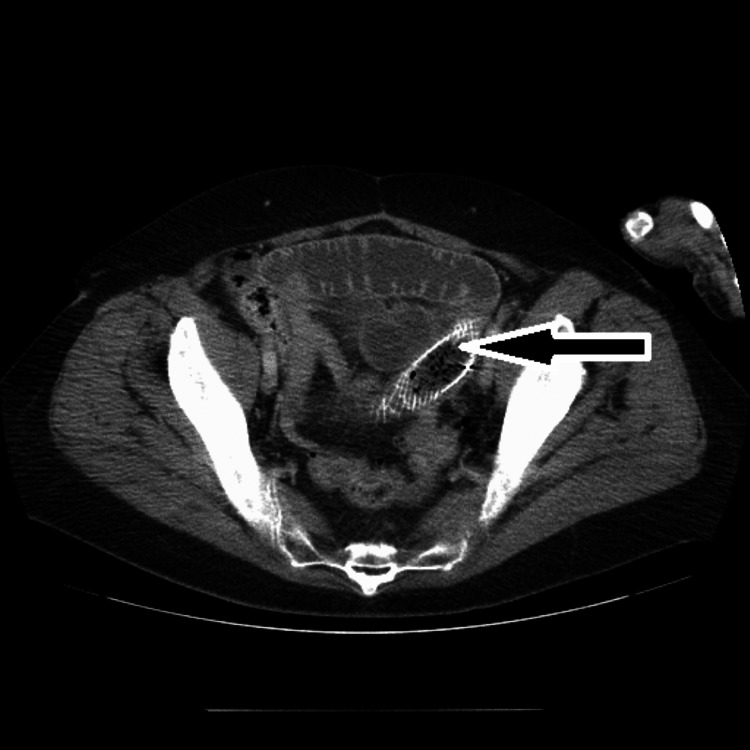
Axial CT demonstrating the stent (black arrow) located within the small bowel with proximal dilation. CT: computed tomography
